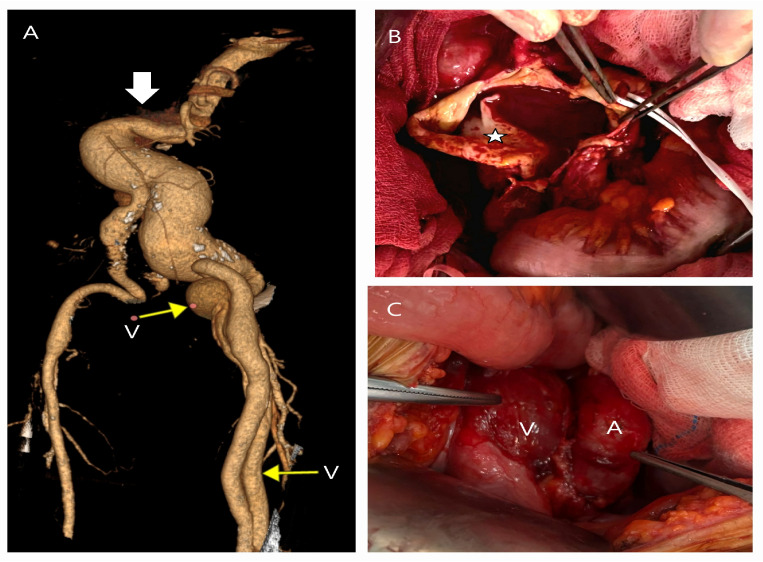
CT and surgical images of an abdominal aortic aneurysm in a 78-year-old male presenting with a pulsating abdominal mass. The patient, a non-smoker with no comorbidities, reported, ’I feel my heart beating in my abdomen’. A 3D CT image (A) shows a neck angulation >60° (white arrow) and venous dilatations (V) from the left popliteal to iliac veins (yellow arrows) due to an arteriovenous fistula. Intraoperative findings (B) revealed a thin-walled aneurysm with chronic partial dissection (white star) but no thrombus. (C) demonstrates severe venous dilation and adhesion between the left common iliac artery (A) and vein (V), complicating surgical exploration.
